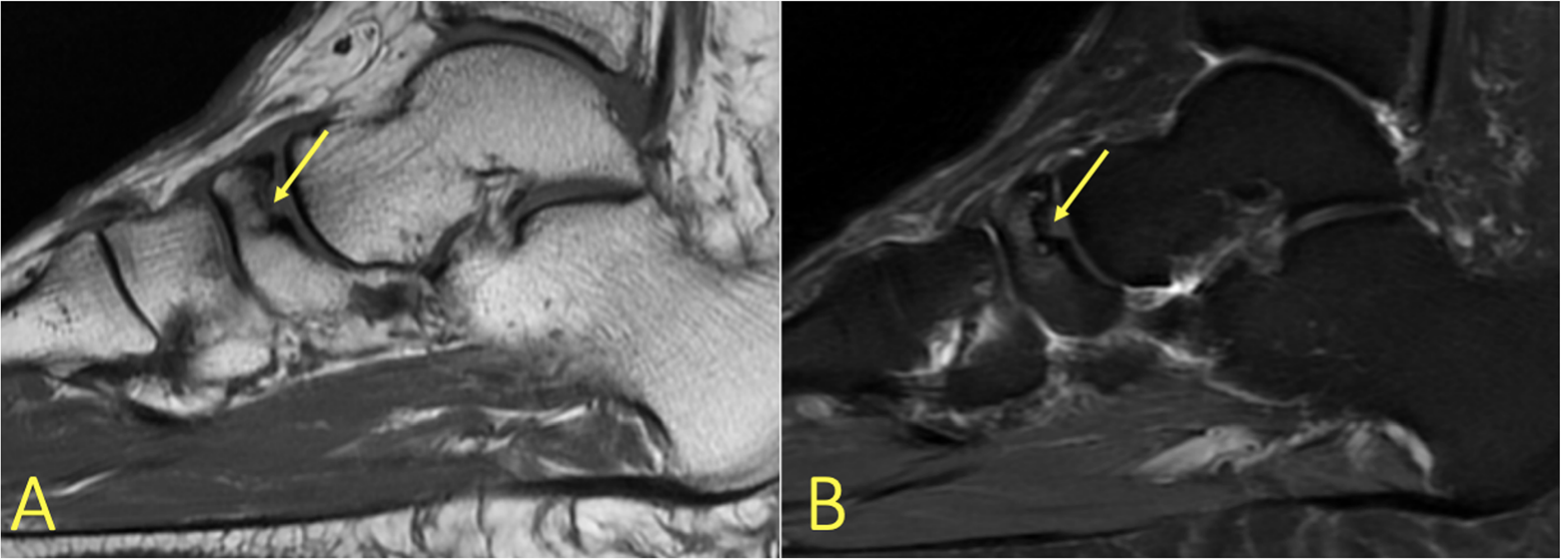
A 61-year-old female with hindfoot pain. MRI showed osteonecrosis of the navicular bone (arrows; Mueller-Weiss syndrome)
